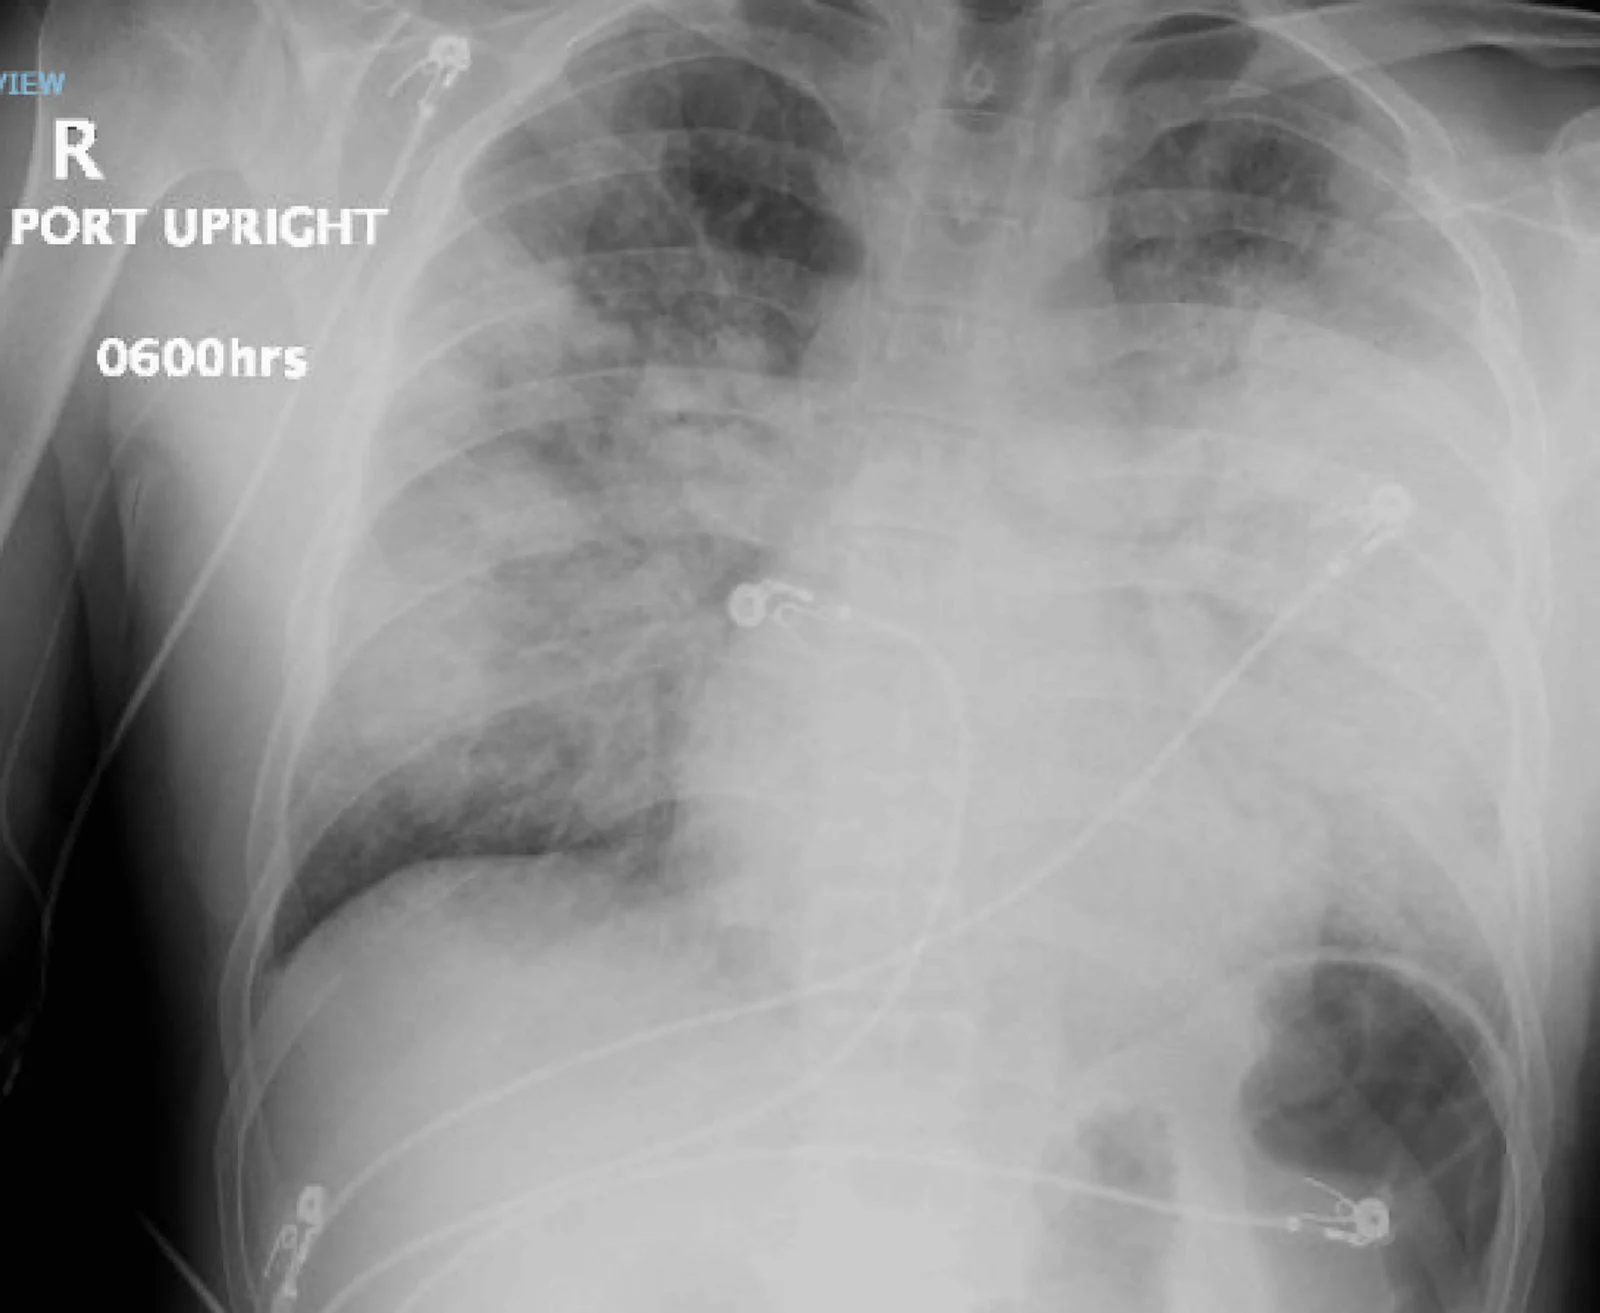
Admission chest radiograph. Portable chest radiograph obtained on admission demonstrating diffuse bilateral patchy air-space opacities, most prominent in the perihilar and upper lung fields, consistent with acute pulmonary edema and acute lung injury. Findings correlate with the patient’s severe hypoxemic respiratory failure and acute cardiomyopathy.
